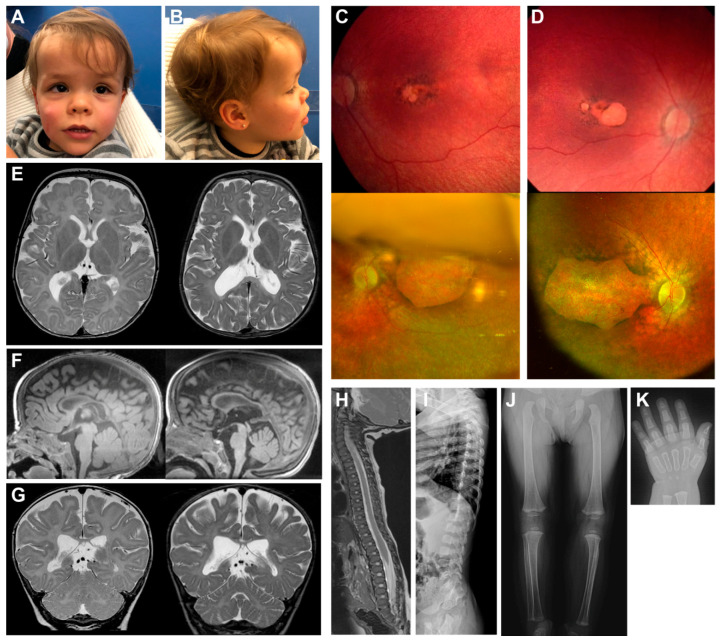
Clinical images of the patient. Frontal (A) and lateral (B) photographs of the patient’s head show facial appearances with mild coarsening and a deep nasal bridge. Retinographies of the left (C) and right (D) eyes present macular colobomatous involvement due to a retinal degeneration. Upper images were obtained at the age of six months whereas lower images were taken at age two years. Cerebral magnetic resonance imaging (MRI) (E–G) show hypomyelinating leukoencephalopathy, and progressive brain and cerebellum atrophy. Left images were obtained at the age of six months, whereas right images were obtained at age two years. Lateral spine MRI image and radiograph (H,I, respectively) present small epiphyseal changes due to a spondylo-epiphyseal dysplasia at six months of age. Skeletal radiographs (J,K) show severe ossification delay when the patient was 20 months old.
